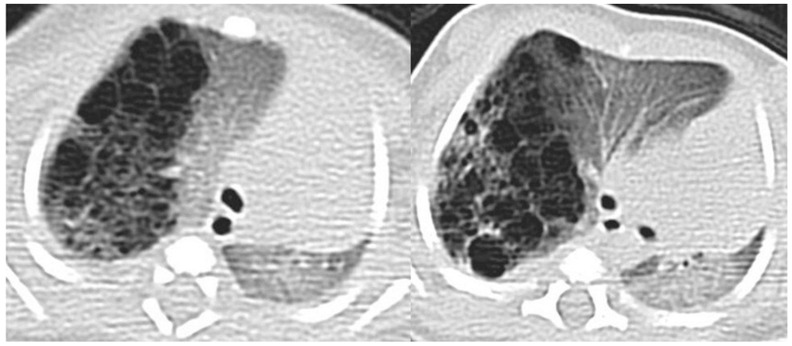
A CT scan later performed in the same patient as in Figure 4, showing multiple small air-filled cysts in the right upper lobe and in the right lower lobe, with a maximum diameter of 20 mm, confirming the diagnostic hypothesis of type 2 CPAM.
